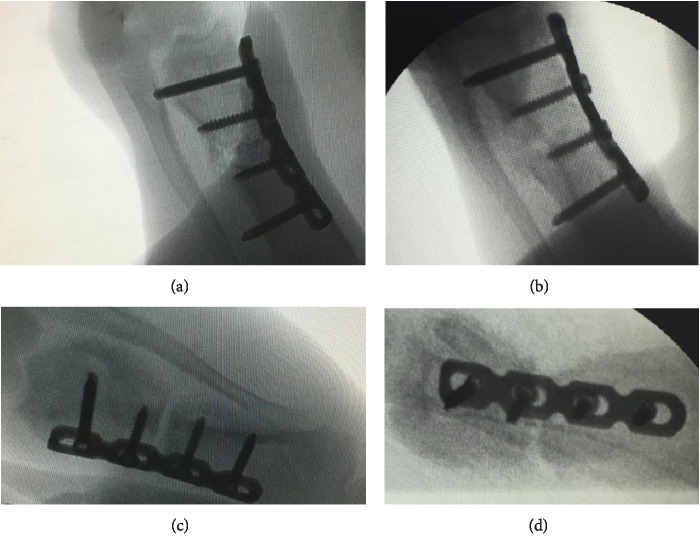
Models of defects B1 and B2 and radiography. Time-dependent radiological changes in the defect, 4 and 8 weeks after reconstruction. (a, b) 4 and 8 weeks after reconstruction, the *Tibia 3* showing bone bridge connecting the defect B1. (c, d) 4 and 8 weeks after reconstruction, the *Tibia 4* showing overgrowth of callus upon bilateral ends aside the defect B2, without crossing the gap.
